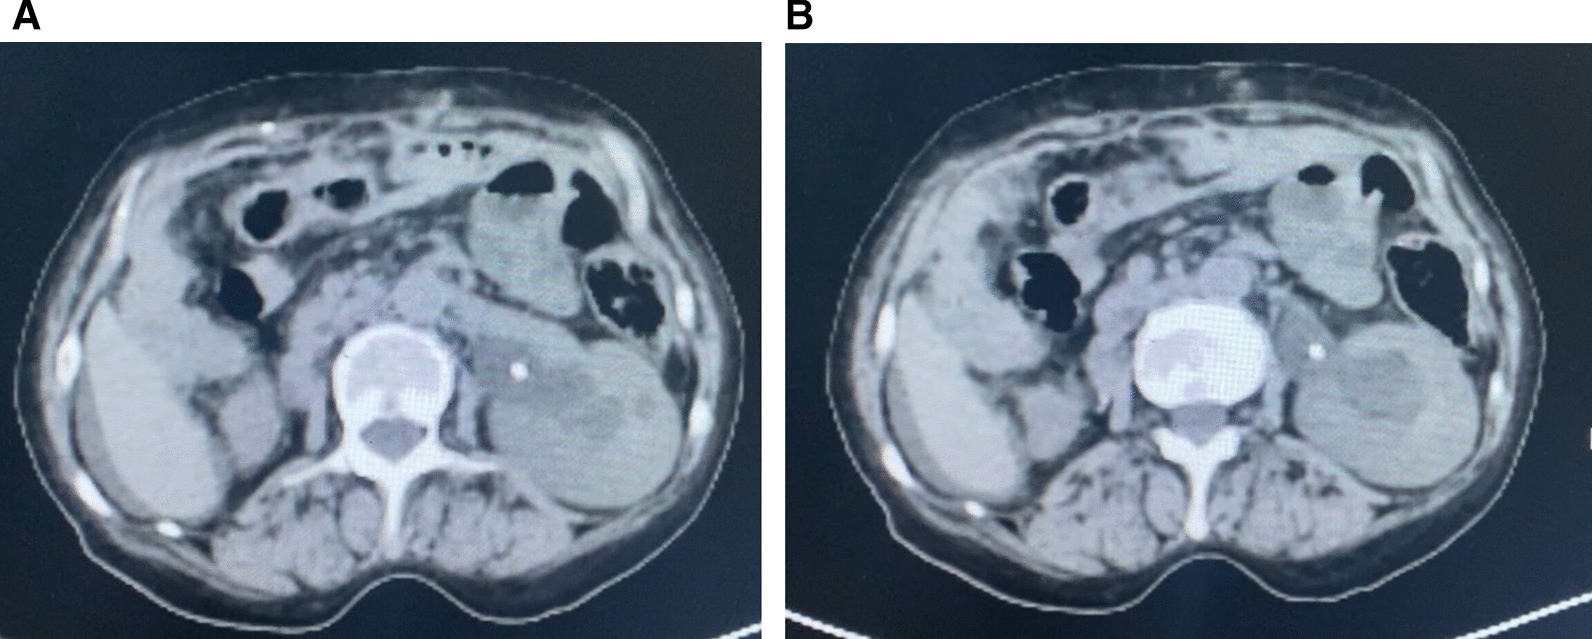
A, B Axial CT scan showing the state of the solitary left kidney with the double J stent in place
